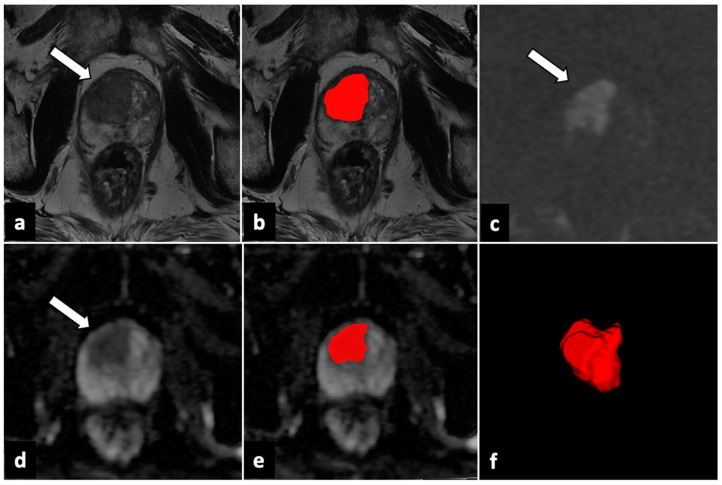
ROI delineation and generated 3D VOI in a transitional zone lesion. In the right transitional zone of the gland apex, an ill-defined lesion with a major diameter >15 mm is observed (*white arrows*). The lesion shows a moderately low signal in the T2W sequence (a), a high signal in DWI (c), and signal loss in the ADC map (d), consistent with a PI-RADS 5 category. It was segmented with T2W and ADC (b,e), and a 3D VOI of the lesion (f) was constructed. ADC: apparent diffusion coefficient; DWI: diffusion-weighted imaging; ROI: region of interest; PI-RADS: Prostate Imaging–Reporting and Data System; T2W: T2-weighted; 3D VOI: three-dimensional volume of interest. Red part shows the contouring and final volume obtained from the lesion.
